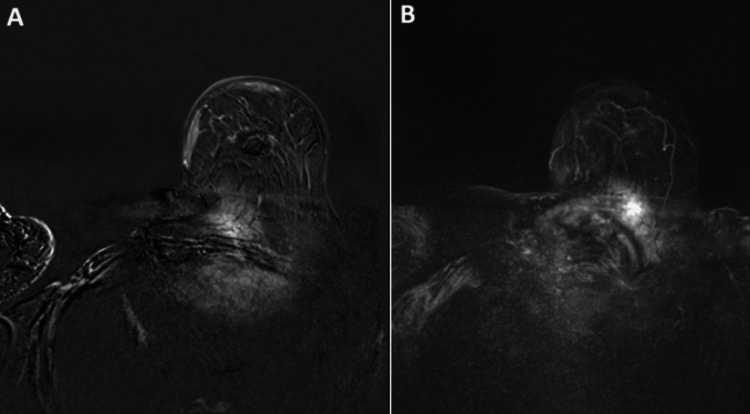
Seven months post-initial ablation. (A) T1 arterial phase showing ablated area with no suspicious abnormal enhancement. (B) Post-contrast dynamic MRI of the breast with MIP subtraction showing the continuous absence of the primary lesion and no residual ipsilateral axillary lymph node metastases after re-ablation MIP: maximum intensity projection
